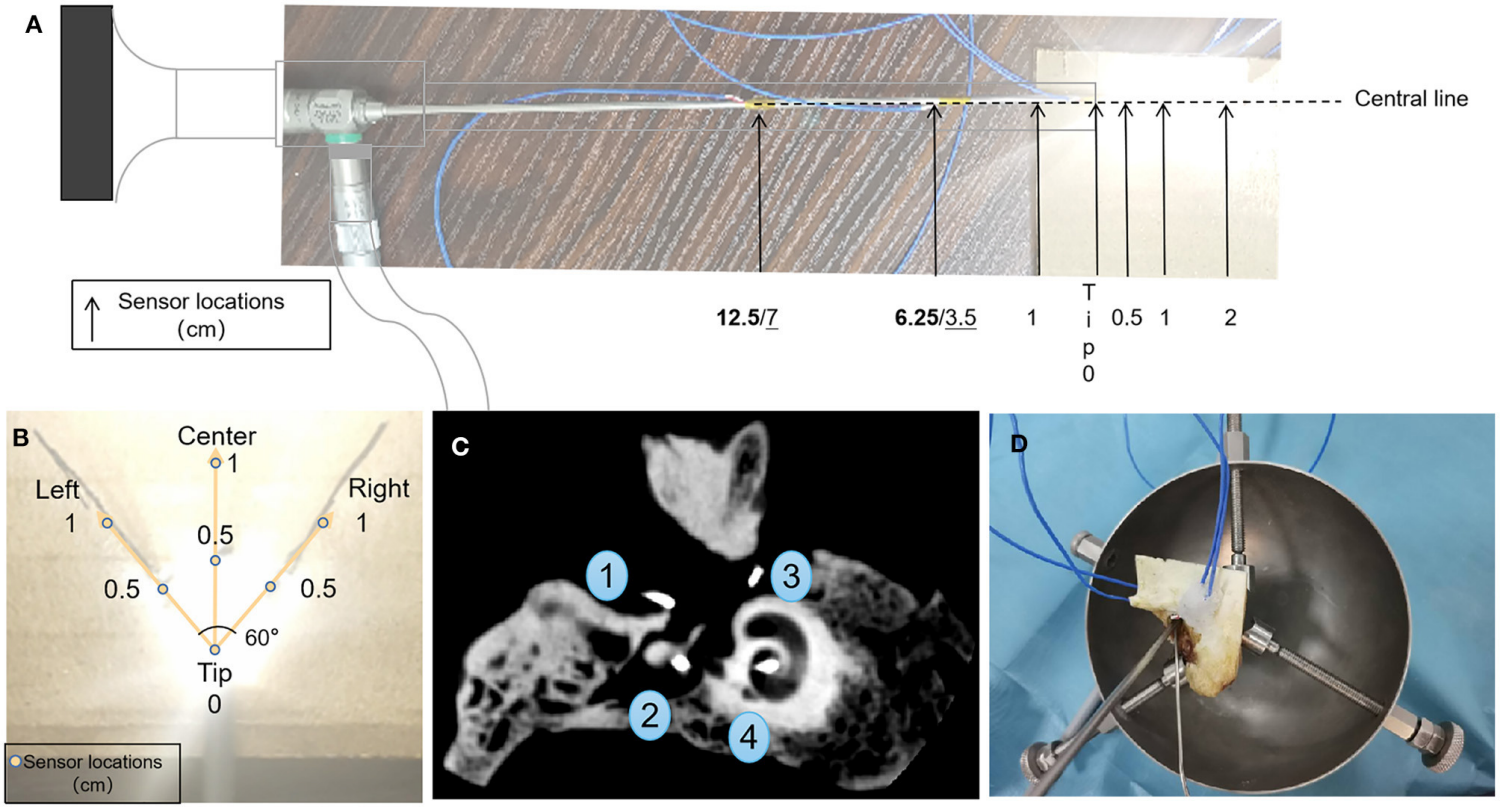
Configurations for sensors in the open environment (A,B) and temporal bone (C), and transcanal endoscopic ear surgery settings (D). (A) Temperature sensors placement relating to the tip of the endoscope. Boldface indicates the 1/2 shaft length and 1/4 shaft length distances (cm) of the sensors to the rear of the tip of the neuroscope, and underline indicates their placement to the rear of the tip of the oto-endoscope. (B) Temperature sensors placement according to the illuminated area. (C) Representative cone-beam computed tomography images of sensor positions in temporal bone 4R. Fundus of the external auditory canal, aditus ad antrum, round window/niche, and modiolus/fundus of the inner auditory canal.
